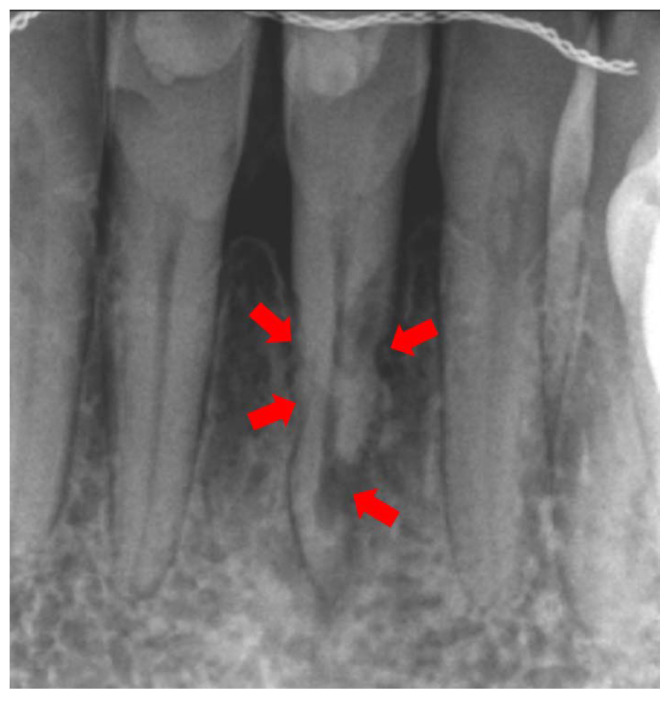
Periapical radiograph of tooth #24. Extensive resorptive defect observed on multiple areas of the dental root. The prognosis for this tooth was deemed guarded due to advanced ERR.
